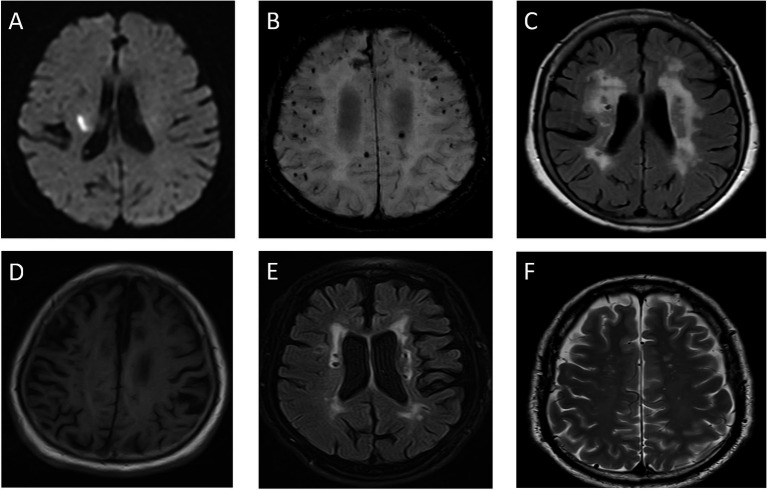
Imaging of cerebral small vessel disease. (A) DWI sequence shows hyperintense lesion in right cerebral hemisphere, indicating acute ischemic lesion. (B) SWI sequence reveals multiple hypointense cerebral microbleeds (CMBs) in brain parenchyma. (C) FLAIR sequence displays hyperintense white matter lesions adjacent to lateral ventricles. (D) T1WI shows brain atrophy with widened sulci and enlarged ventricles. (E) FLAIR sequence demonstrates small patchy hyperintense lacunar infarction lesions. (F) T2WI shows dilated perivascular spaces (VRS) as small round hypointense foci.
